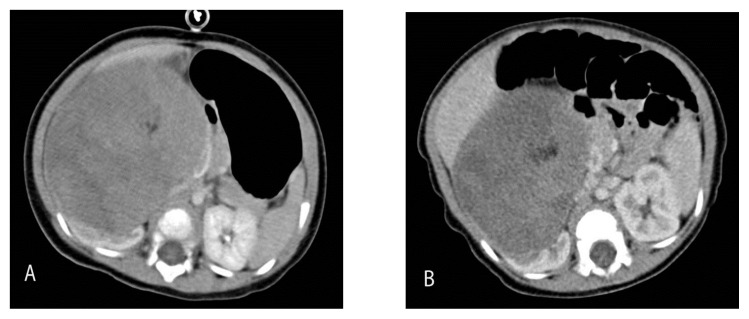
CT abdominal scan of a 14-month-old child: (A) at the time of diagnosis using 100 kVp/100 mAs and (B) at 4 months follow-up using 100 kVp/50 mAs. The calculated EDs for these examinations were: (A) 12.9 mSv and (B) 6.5 mSv. With the lower effective charge, the image remains diagnostic, even though it is grainier, and most importantly it resulted in significant dose reduction
